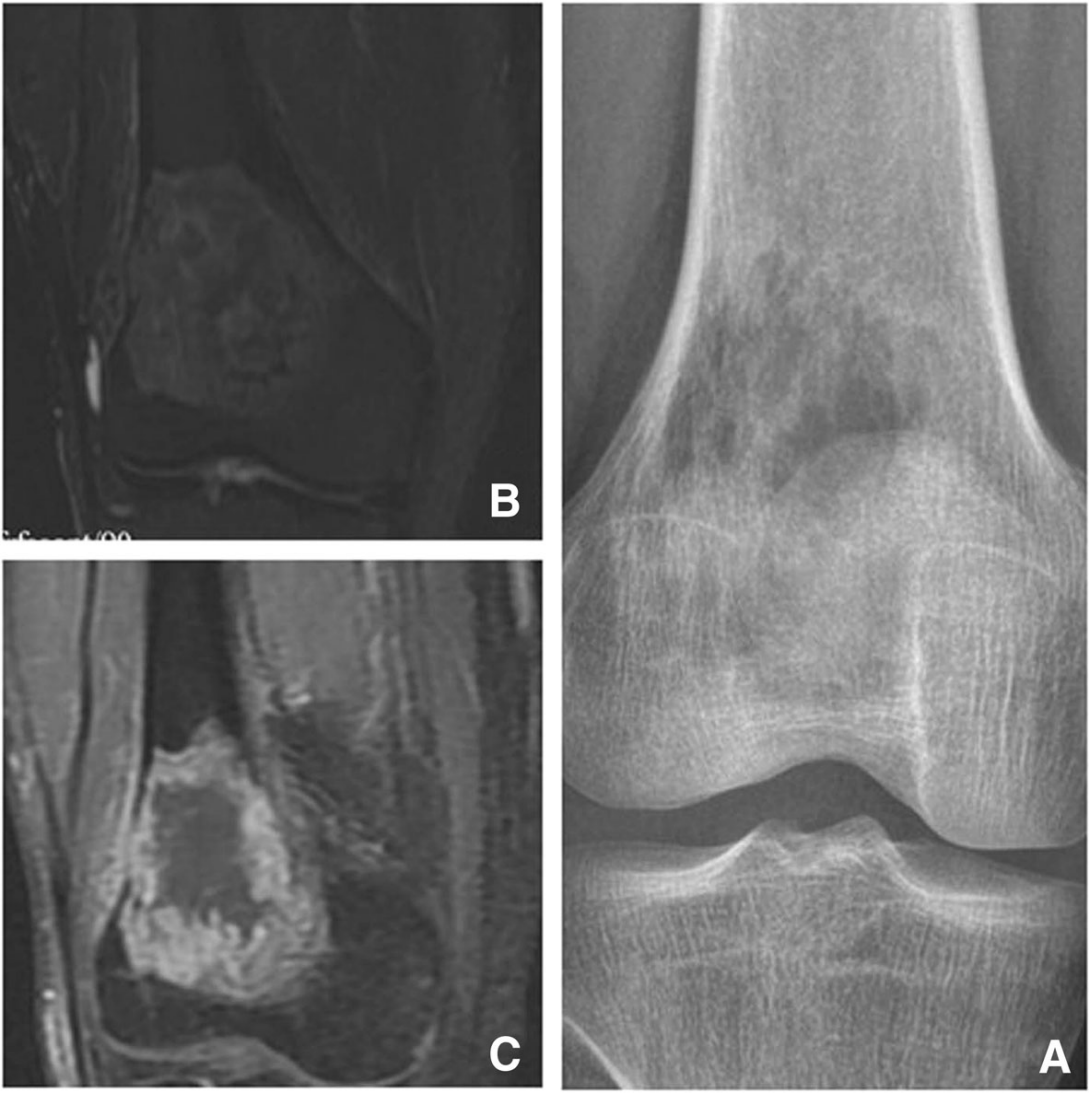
LGMS of the right shoulder. An irregular soft tissue mass in right shoulder with equal T1 and long T2 was observed (a, b). Enhanced MRI revealed a homogenous mass (c)
